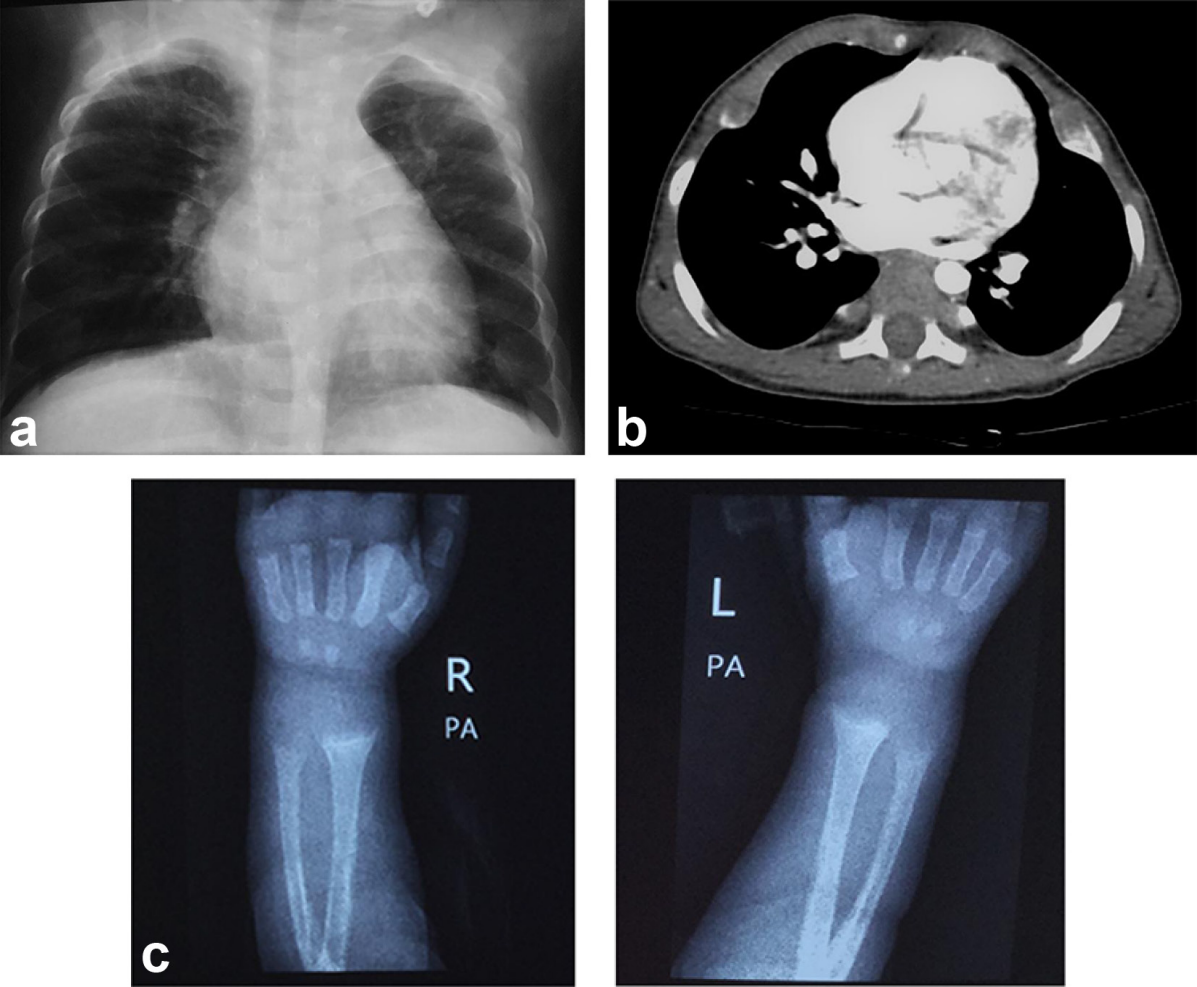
(a) Chest radiograph anteroposterior view, expansion of the anterior ends of ribs is clearly evident, particularly in the left lower ribs. (b) CT scan chest axial view also shows expansion of anterior ends of ribs consistent with rachitic rosary.(c) X-ray of both wrist joints. There is loss of provisional zone of calcification with widening of growth plate. Metaphysis appears flared and fraying is also evident. All these findings point towards rickets.
